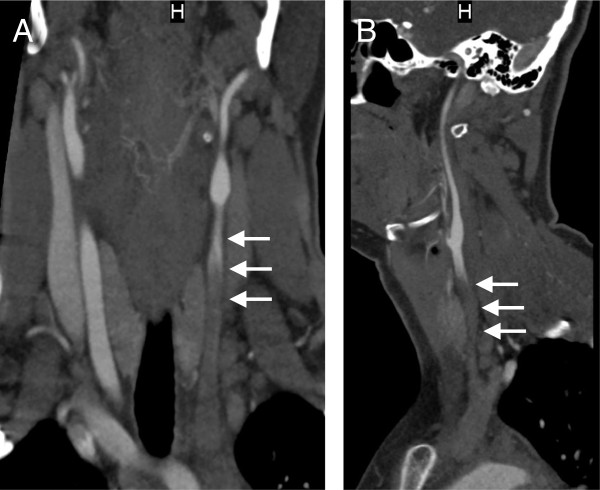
CTA of the neck immediately following CVA. Anterior (A) and lateral (B) views of a CT angiogram of the neck demonstrating thrombosis of the proximal and mid left common carotid artery (arrows).
